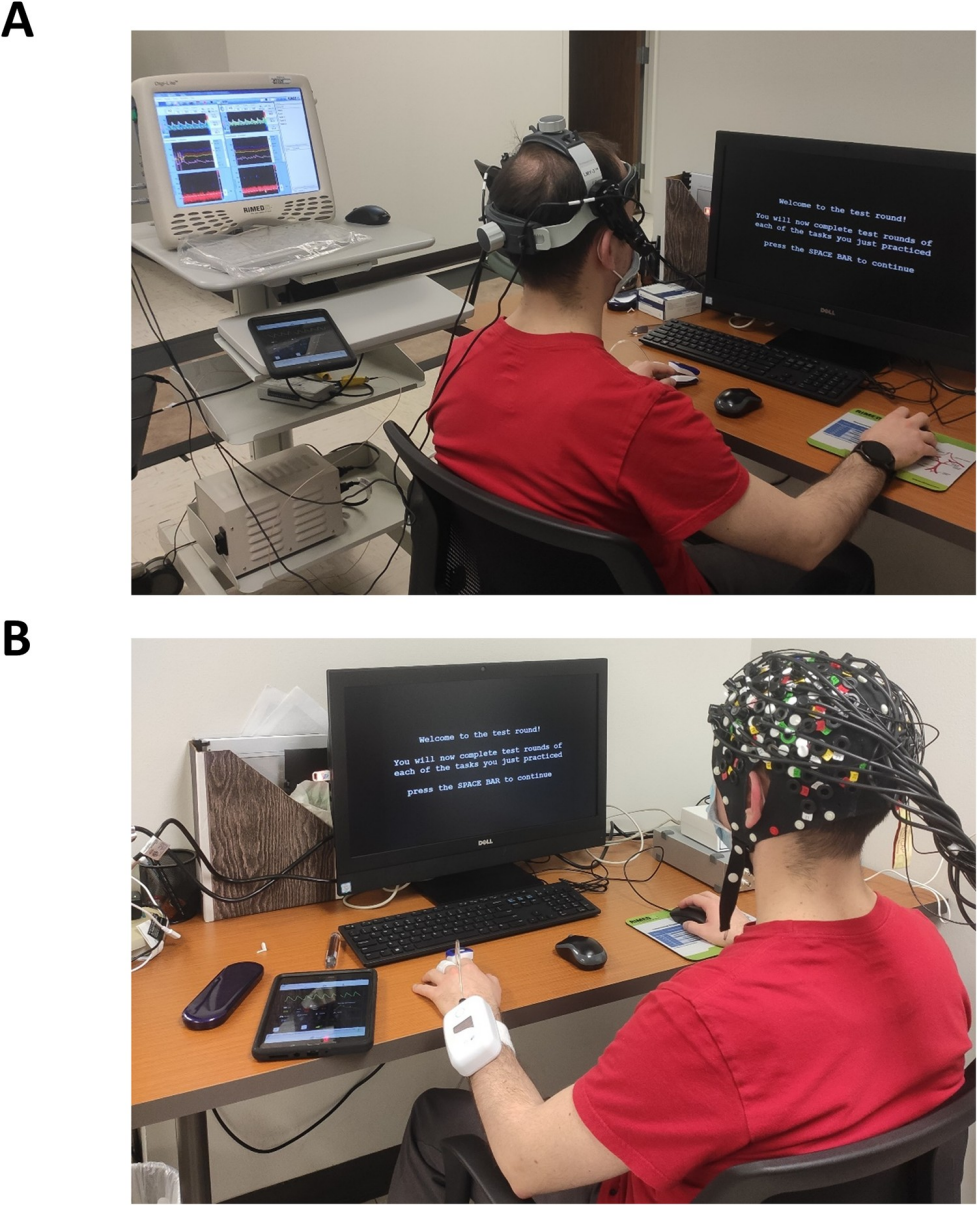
Experimental setup. Participants were seated in front of the computer that delivered the cognitive paradigm. Changes in cerebral hemodynamics were recording during cognitive stimulation with transcranial Doppler sonography (Panel A) and functional near-infrared spectroscopy (Panel B).
